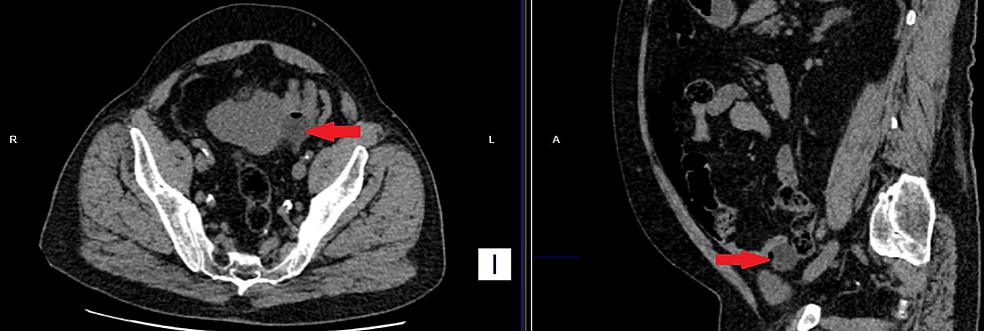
Non-contrast CT To the left, the axial cut view shows the inflated catheter balloon (red arrow) outside the bladder. To the right, the sagittal cut view shows the balloon (red arrow) out of the bladder.
